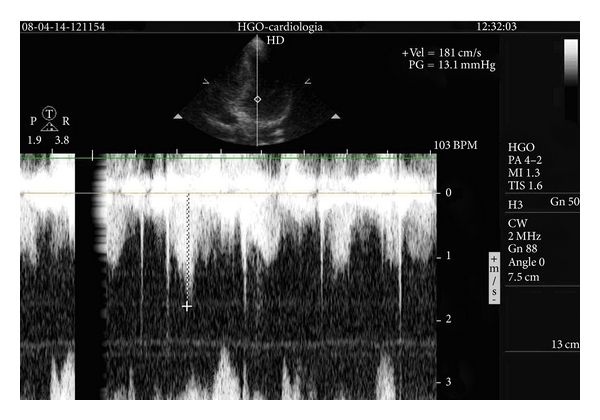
Flow evaluated with continuous wave Doppler at peak exercise demonstrating the absence of LVOT obstruction.
